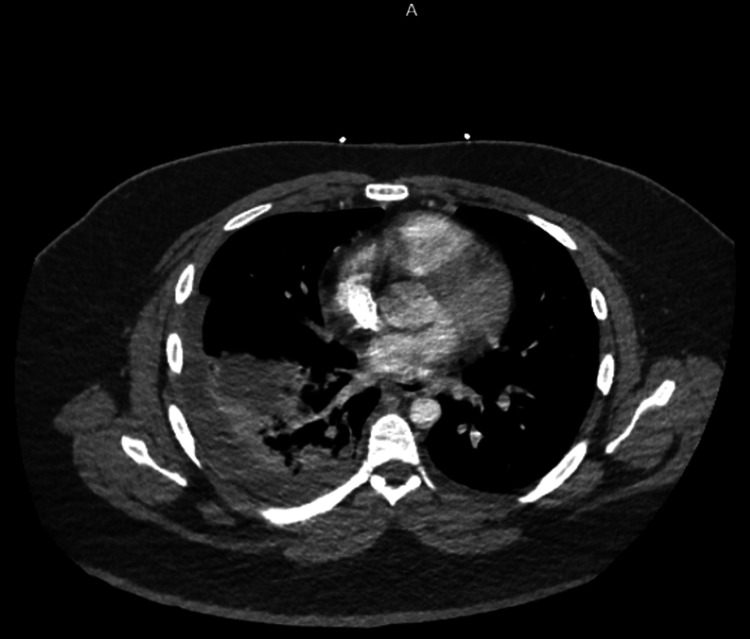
Chest CTA showing lobulated moderate left pleural effusion with consolidation in the right lower lobe, along with a mass-like consolidation in the superior segment of the right lower lobe CTA: Computed tomography angiography
